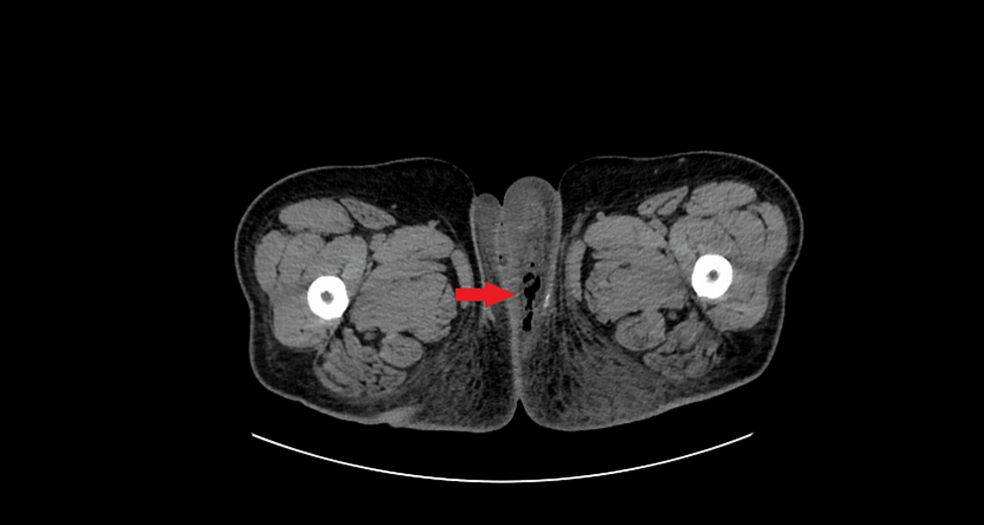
CT scan of the lower abdomen. The arrow points to the presence of free air in the subcutaneous tissue.
